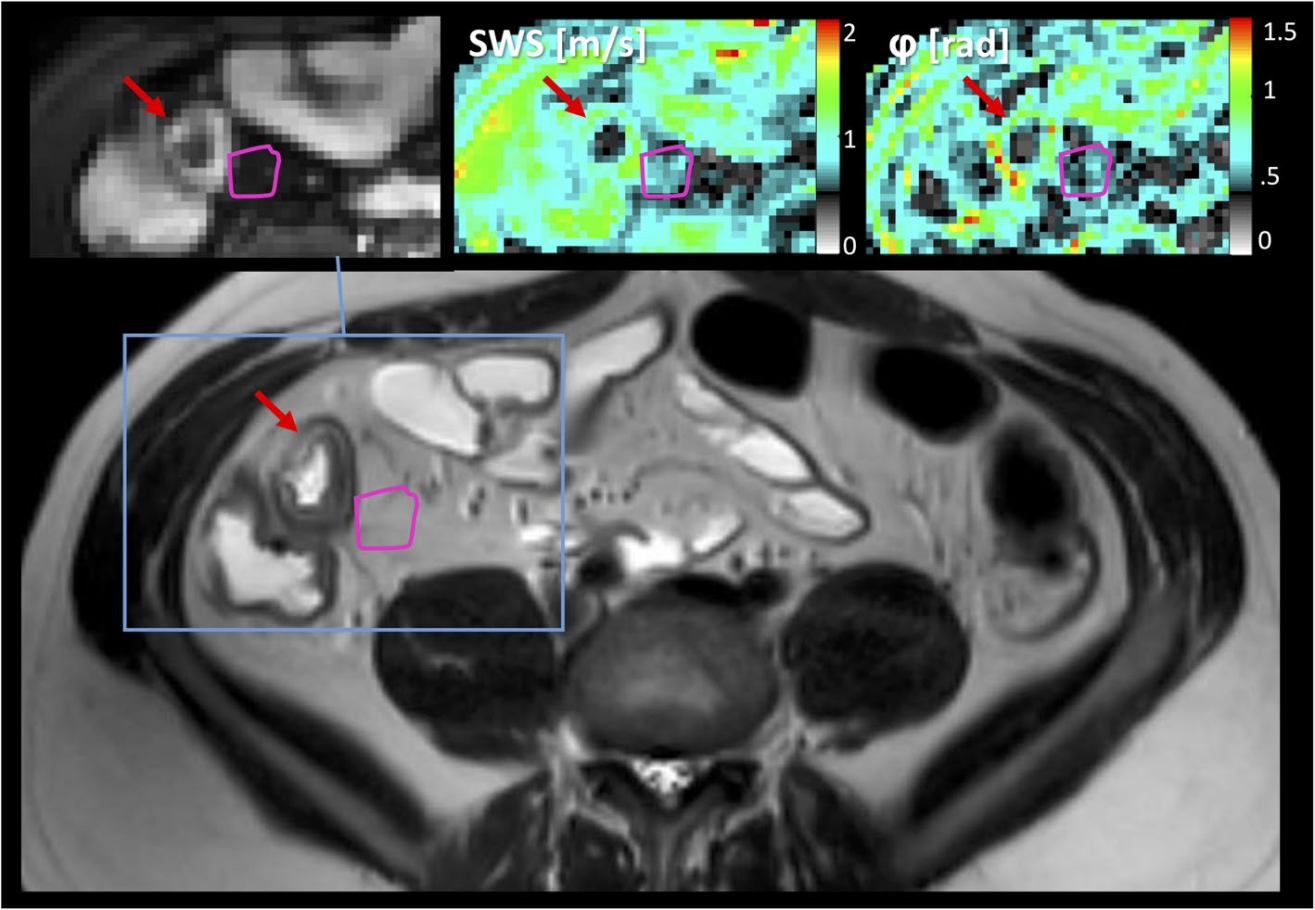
Region of interest of affected bowel mesentery in a representative patient with Crohn’s disease on T2-weighted images, on top in the inset is the corresponding magnitude magnetic resonance elastography image with the shear wave speed (SWS) and phase angle (φ) map. Segmented affected mesentery is highlighted in pink, and the red arrow shows the affected bowel wall
